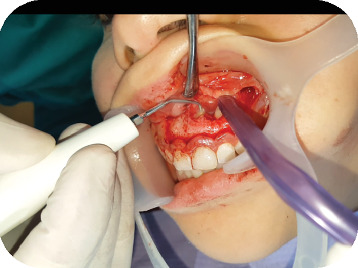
Retrograde preparation with ultrasonic insert Acteon AS6D.
